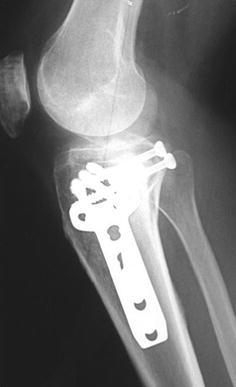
Schatzker type V fracture, 4 months X-rays (LL)
